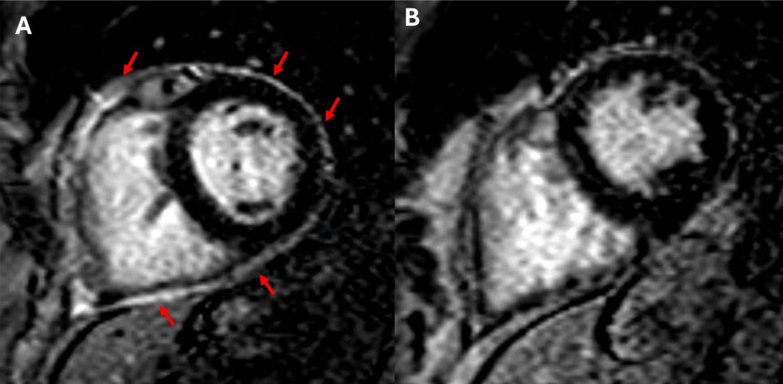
Case Presentation #2 A 66-year-old female diagnosed with pericarditis post pacemaker implantation. Figure A is a short-axis slice of cardiac magnetic resonance imaging showing severe late gadolinium enhancement (arrows). Figure B is repeat imaging in the same patient 1 year later with improved inflammation after completion of treatment with NSAIDs and colchicine. NSAIDs = nonsteroidal anti-inflammatory drug.
